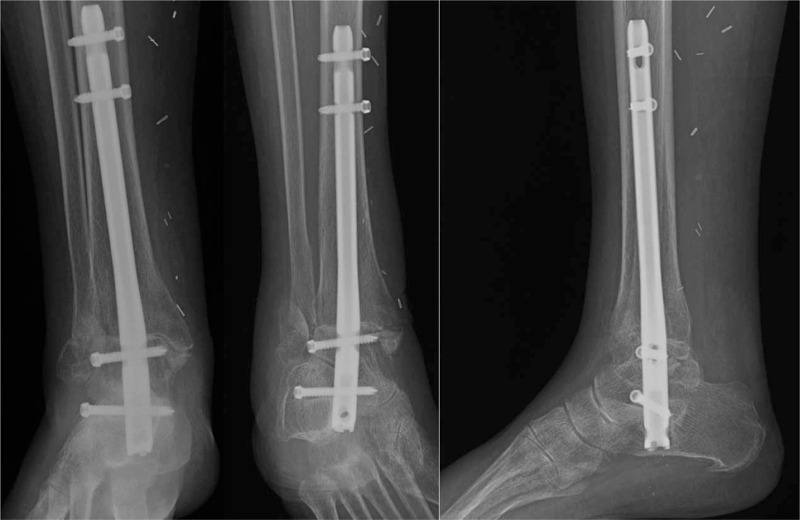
Patient underwent fixation with a tibiotalocalcaneal nail and allowed early weight-bearing. At 3.5months postoperatively, radiographs reveal stable hardware and healing lateral and medial malleolus fractures.
